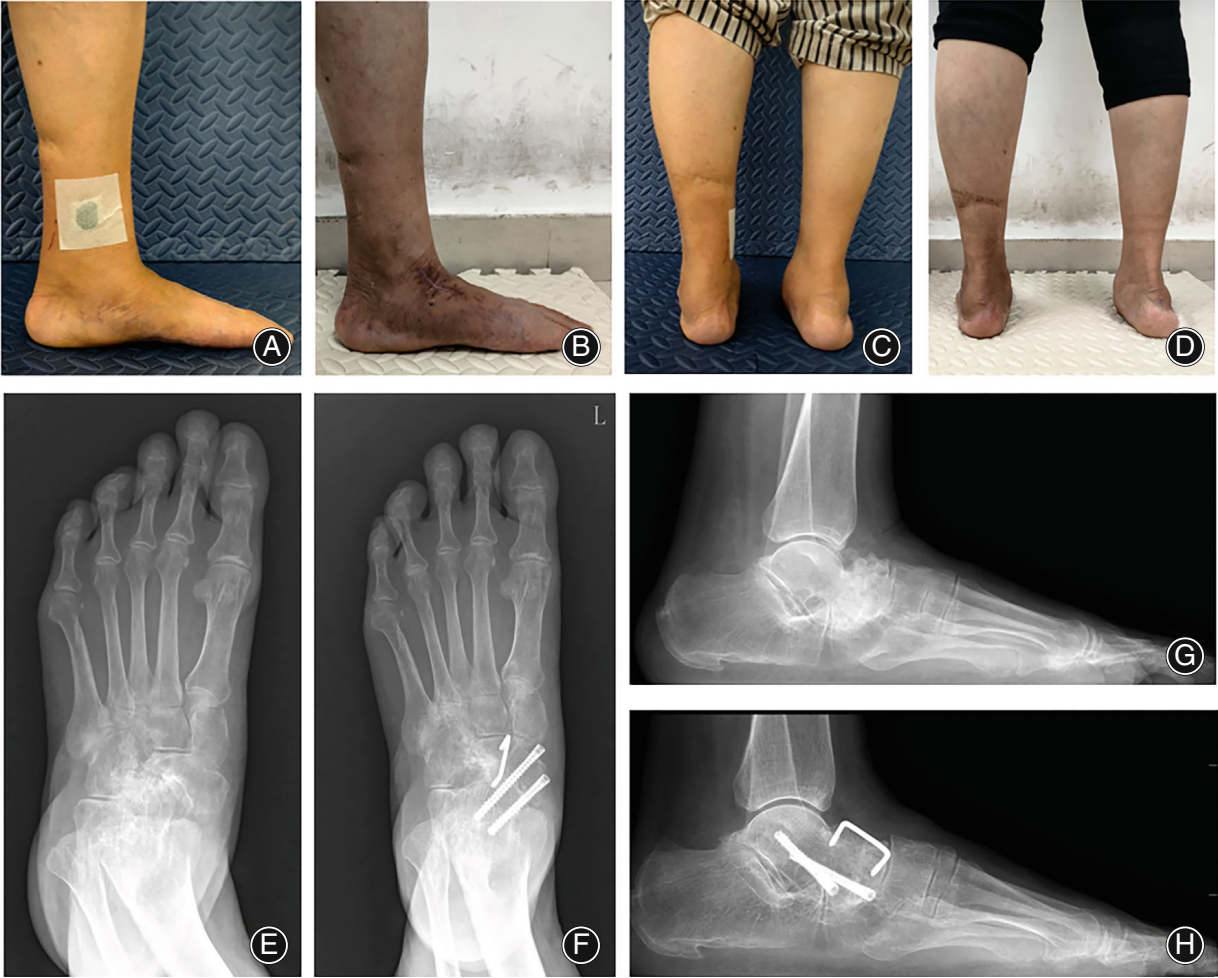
(A,C) preoperative appearance image; (B,D) postoperative appearance image; (E,G) the lateral and anteroposterior (AP) weight‐bearing radiographs of foot preoperatively; (F,H) the lateral and AP weight‐bearing radiographs of foot postoperatively. The force line was corrected.
